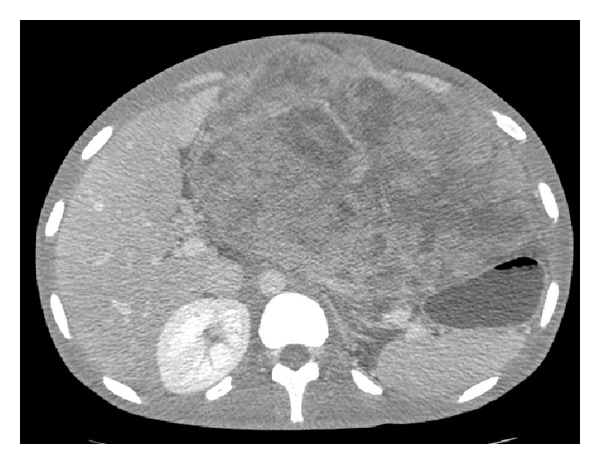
Preoperative CT image revealing a single massive tumor (30 × 21 × 13 cm).
